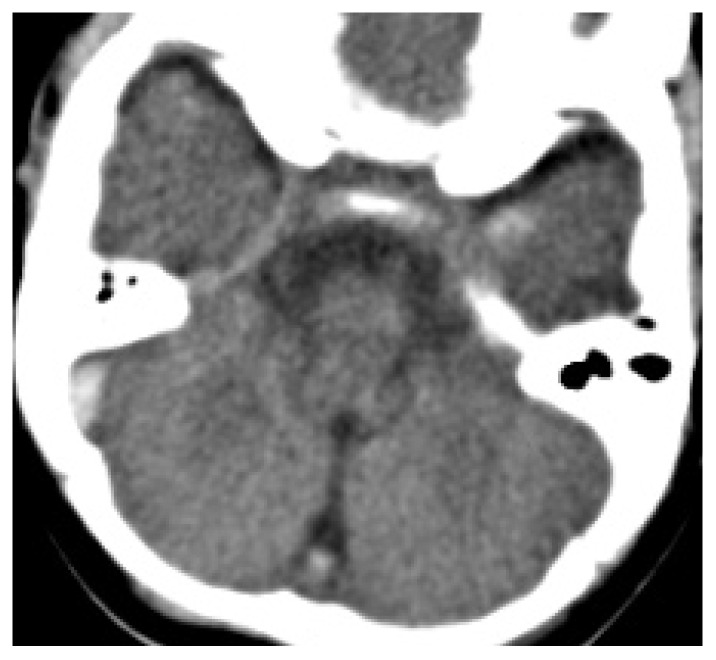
Nonenhanced CT. Spontaneous hyperdensity of the thrombus in the right lateral/sigmoid sinus junction.
